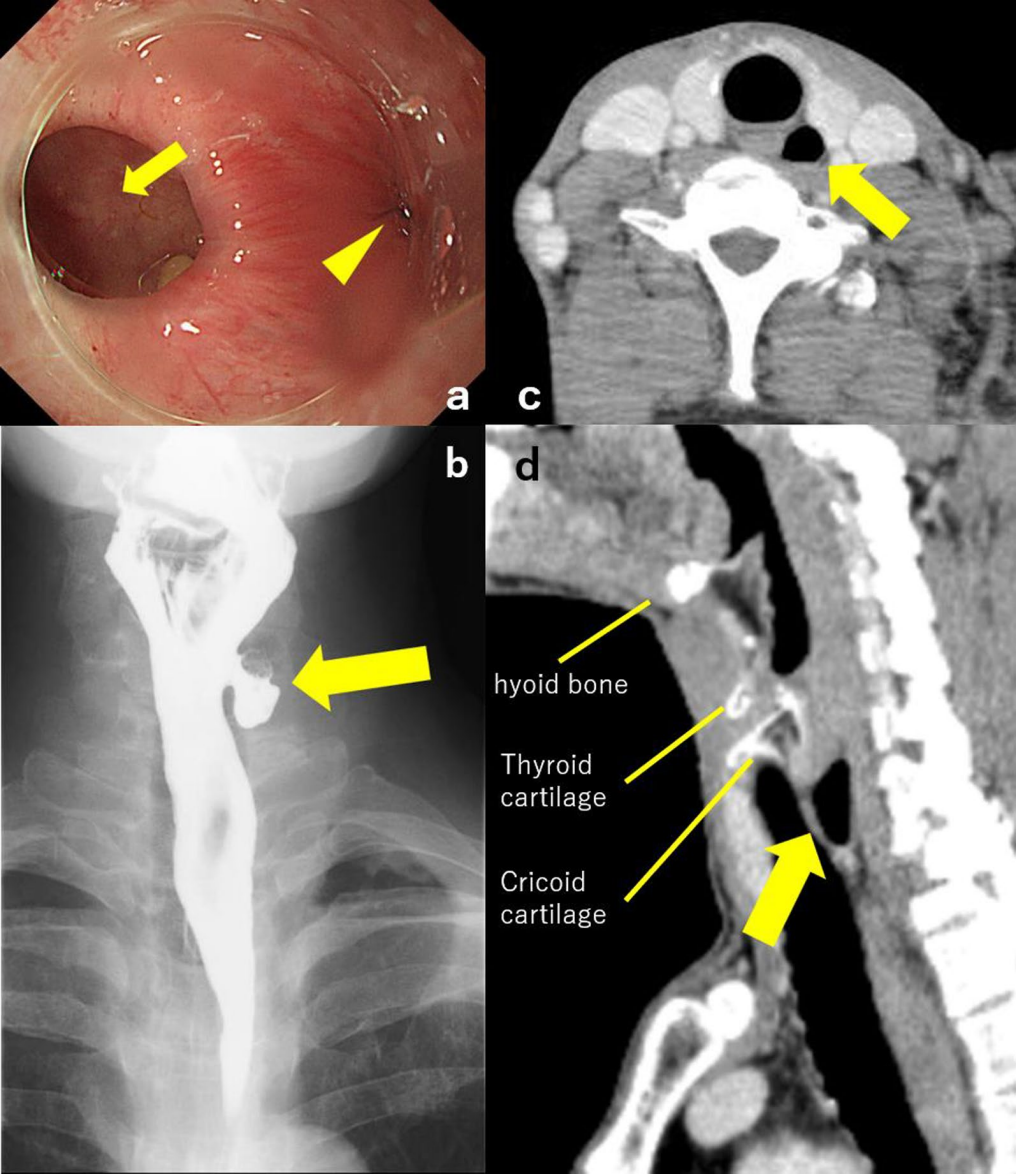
Preoperative findings. a Esophagogastroduodenoscopy showed a diverticulum (arrow) and the true lumen of the esophagus (arrowhead). b Esophagogram revealed the diverticulum at the left side of cervix (arrow). Axial (c) and sagittal (d) section of computed tomography showed an air-contained diverticulum arising from behind the left lobe of the thyroid (arrow). The diverticulum was diagnosed as Zenker’s diverticulum
